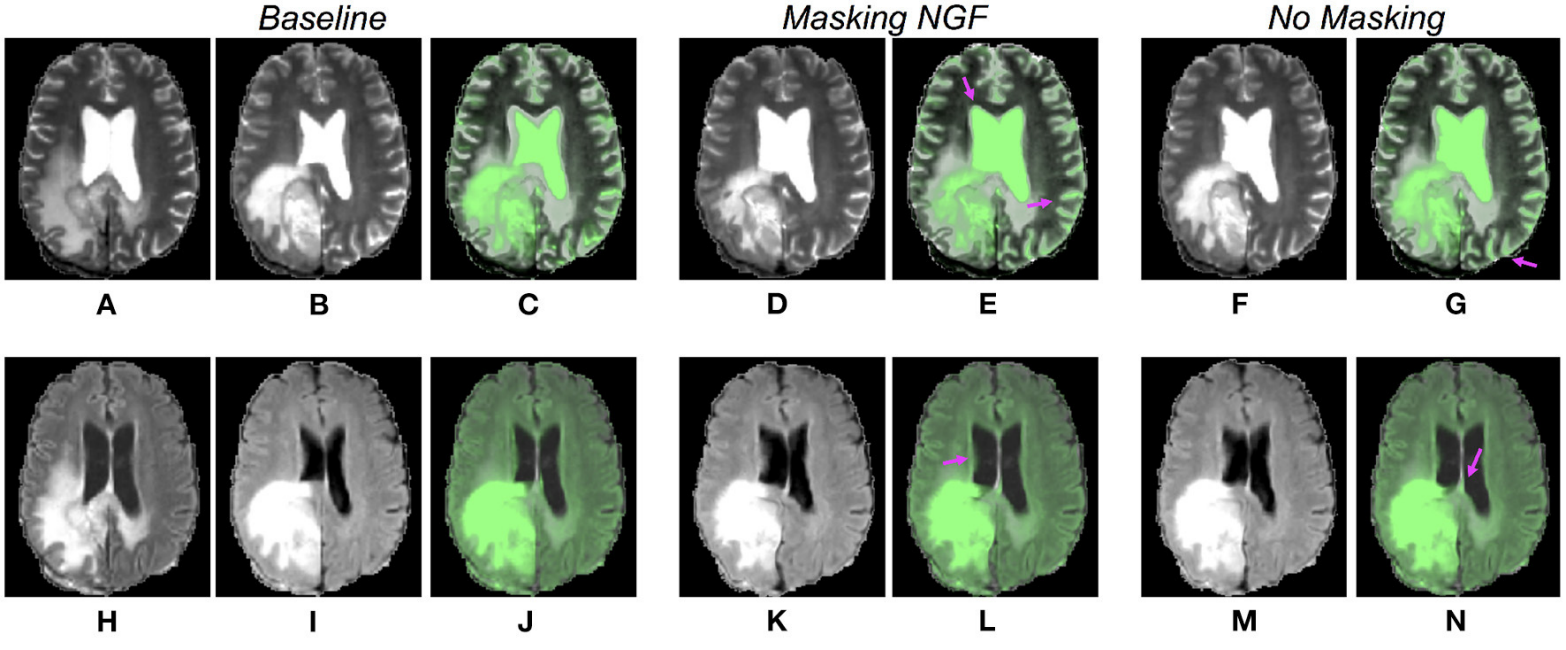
Comparison of qualitative results for CNN models trained on T2 and FLAIR MRI sequences. Each row refers to the results for the CNN models trained on different sequences (from the top to the bottom, T2 and FLAIR). The post- and pre-operative images are visualized in the first and second columns (A,B,H,I), and the initial overlay between the two cases in the third column (C,J). The last four columns display the warped moving volumes (D,F,K,M) and the overlays between the fixed image (post-operative) and the warped moving images (E,G,L,N), respectively, for the models excluding and including the pathological tissues in the distance measure. The purple arrows point to locations where improvements are observable. In panels (E,L,N), a better registration of the lateral ventricles is visible. Besides, in panels (G,E), the sulci are more aligned.
